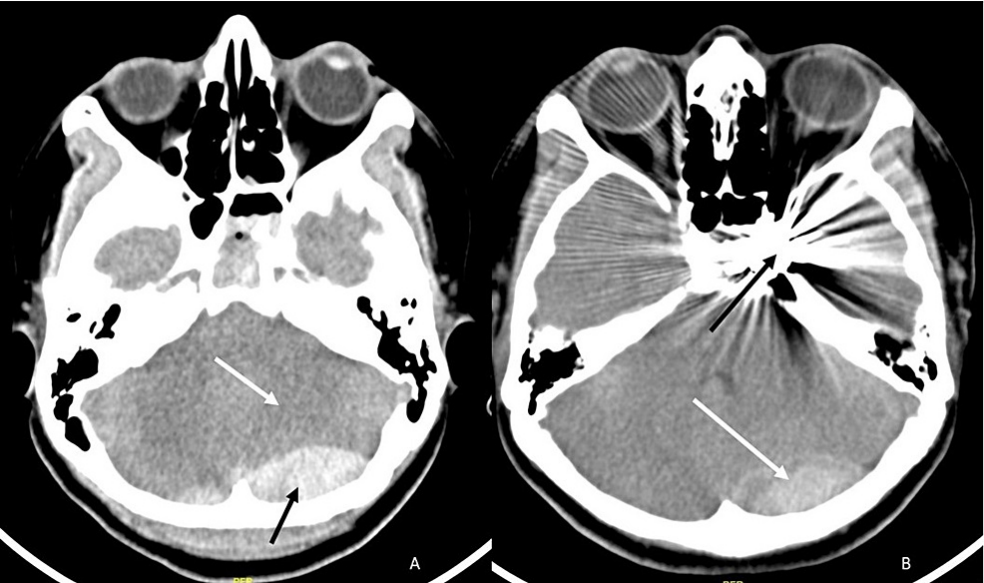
Computed tomography of the brain (A) Acute extradural hematoma is noted in the left occipital region (black arrow) with a mass effect on the left cerebellar hemisphere (white arrow). (B) Post-embolization of the carotico-cavernous fistula shows streak artifacts due to coil mass and embosylate cast (black arrow). The extradural hematoma (white arrow) has not increased in size.
